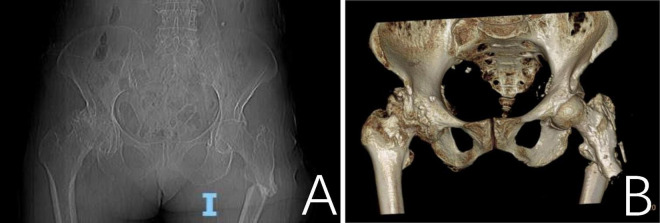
A CT scan revealed a fracture of the proximal segment of the left femur with misalignment and overlapping of the fracture ends. (A) Thickening of the cortical bone in the mid-to-proximal segment of the left femur with irregular margins; a linear, bone-like hyperdense shadow is observed laterally. The medullary cavity shows unevenly increased bone density. (B) The three-dimensional CT reconstruction of the proximal left femur demonstrating fracture morphology.
